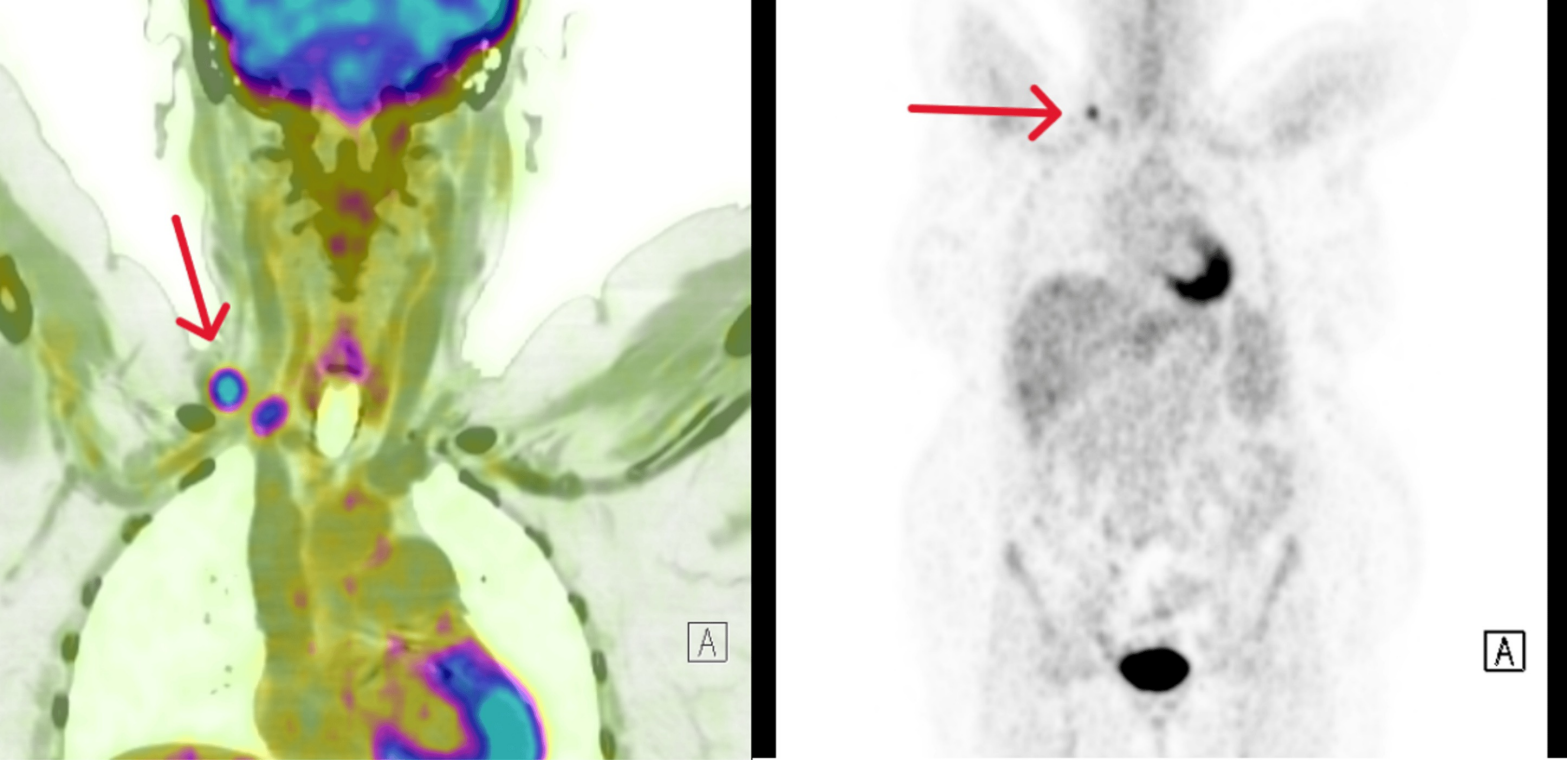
PET-CT scan of the whole body showing FDG uptake (SUV 11.23) in an isolated right supraclavicular lymph node (12 x 9 mm), with no significant uptake observed elsewhere. PET-CT: positron emission tomography-computed tomography; FDG: fluorodeoxyglucose; SUV: standardized uptake value
